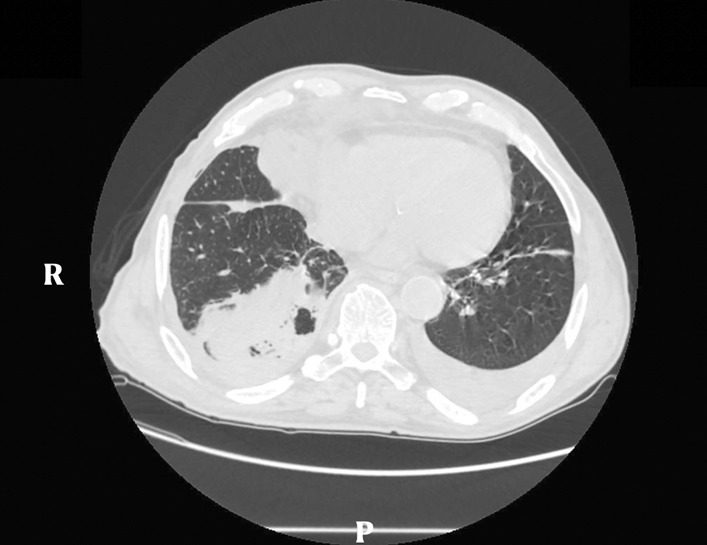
Computed tomography scan of the chest showing a right pleural effusion
